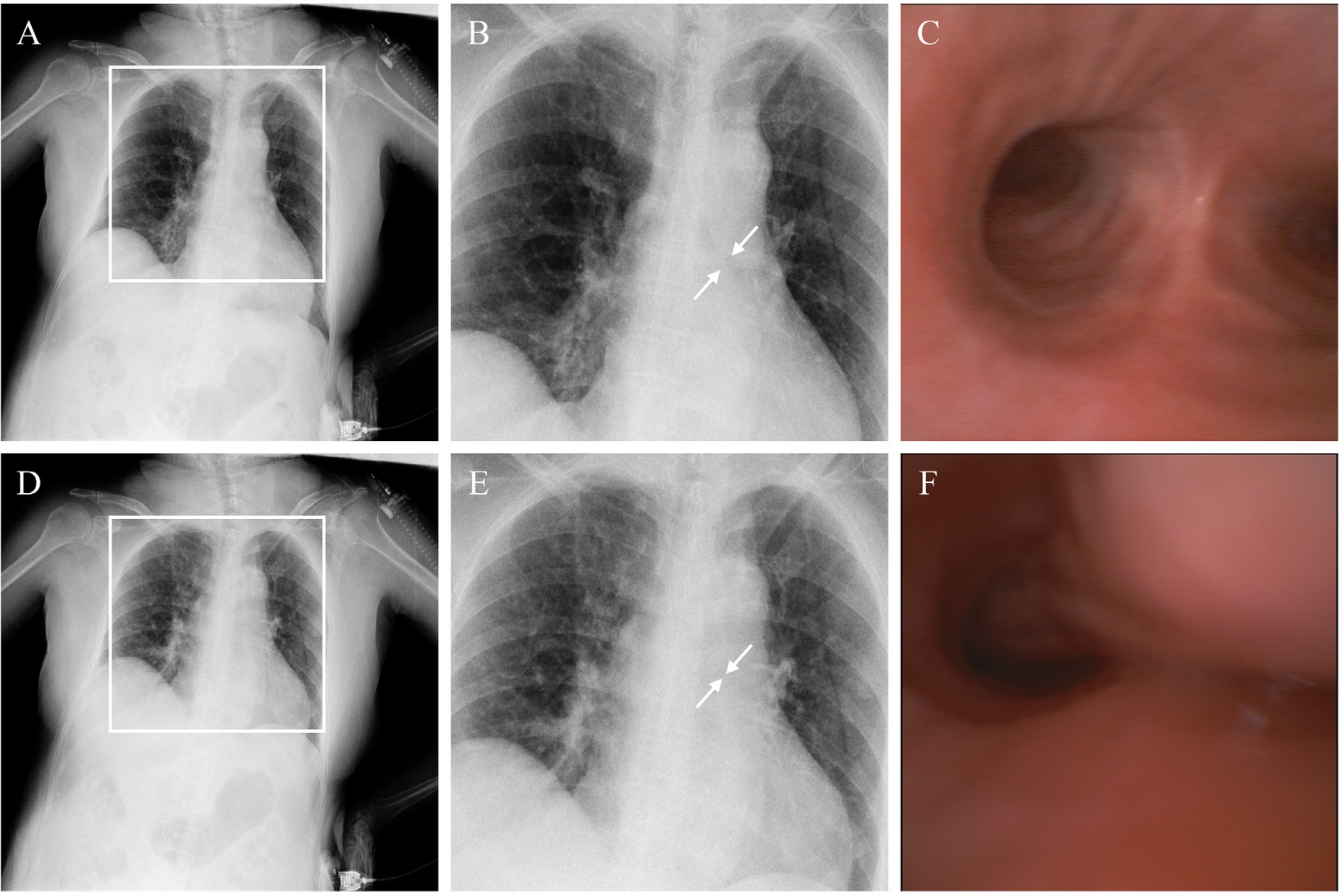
Dynamic chest radiography and bronchoscopic findings in a patient with tracheomalacia. At maximum inspiration, dynamic chest radiography (A) and the magnified chest radiograph (B) clearly show the patency of the left main bronchus (arrow). Bronchoscopy at maximum inspiration (C) also demonstrates patency of both main bronchi. At maximum expiration, dynamic chest radiography (D) and the magnified chest radiograph (E) reveal collapse and obscuration of the left main bronchus (arrow). Bronchoscopy at maximum expiration (F) confirms this collapse. These findings support the diagnosis of tracheobronchomalacia with dynamic airway narrowing during expiration.
